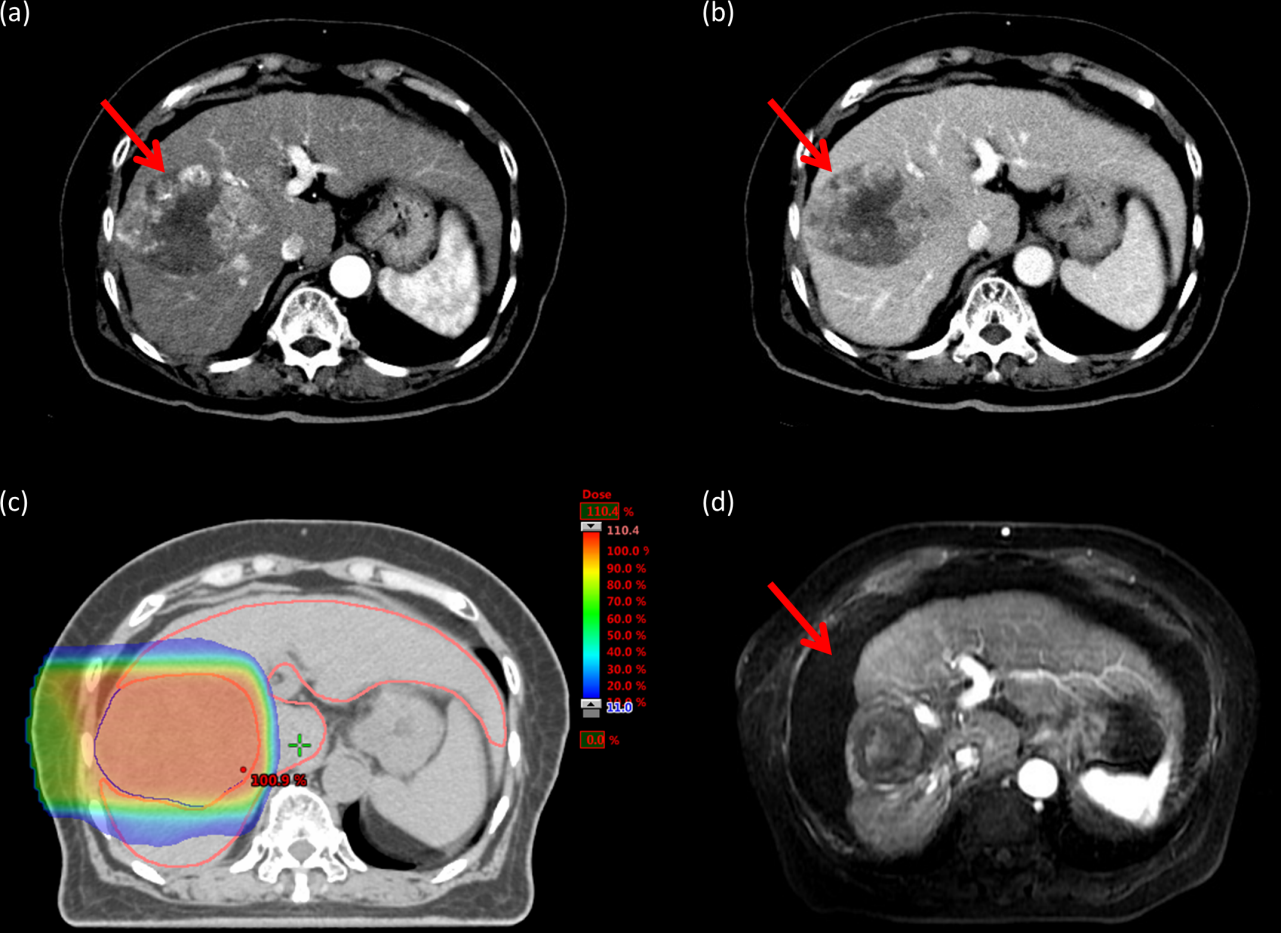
Tumor response of hepatocellular carcinoma diagnosed using imaging and alpha-fetoprotein (AFP) level. Pretreatment alpha-fetoprotein level of this patient was 86762.3 ng/mL. a. Arterial phase of the pretreatment dynamic computed tomography (CT) scan showing enhancement in the primary tumor (red arrow). b. The venous phase of the pretreatment dynamic CT scan showing early wash-out in the primary tumor (red arrow). c. The patient underwent proton beam therapy (PBT). d. Magnetic resonance imaging with contrast 25 months after PBT. Shrinkage and loss of tumor viability of the primary tumor (red arrow).
